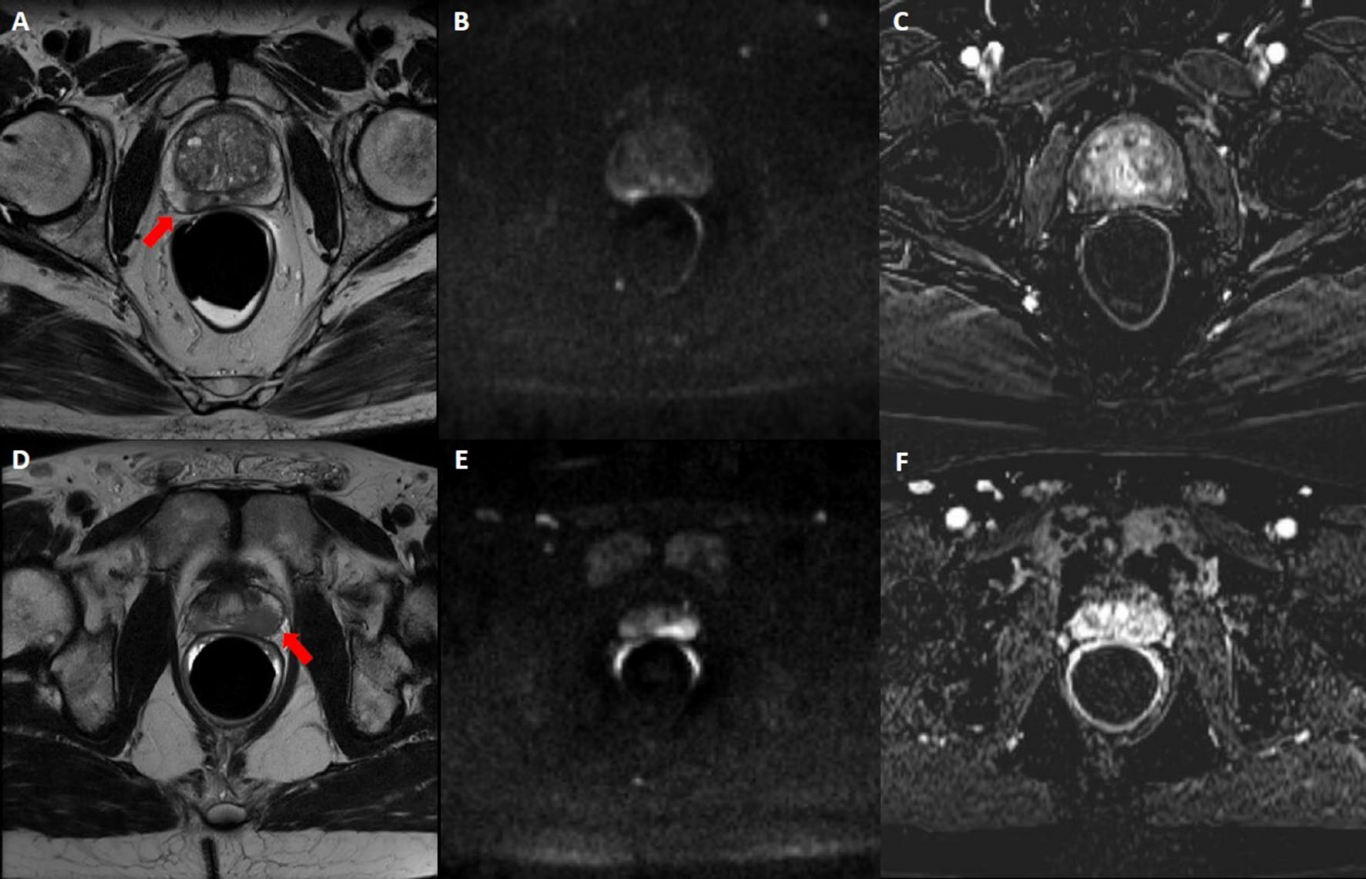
upPI-RADS 4 scored alterations. In the first row, an example of upPI-RADS 4 lesion confirmed at biopsy as GS 4 + 4: mpMRI showed reduced T2 signal intensity in the right PZ (A), altered diffusivity at b1000 DWI (B) with focal contrast enhancement (C). In the second row, an example of upPI-RADS 4 lesion classified as inflammatory at biopsy: mpMRI showed reduced T2 signal intensity in the right PZ (D), altered diffusivity at b1000 DWI (E) with contrast enhancement (F). *PI-RADS* prostate imaging-reporting and data system, *mpMRI* multiparametric MRI, *DWI* diffusion weighted MRI, *PZ* peripheral zone.
